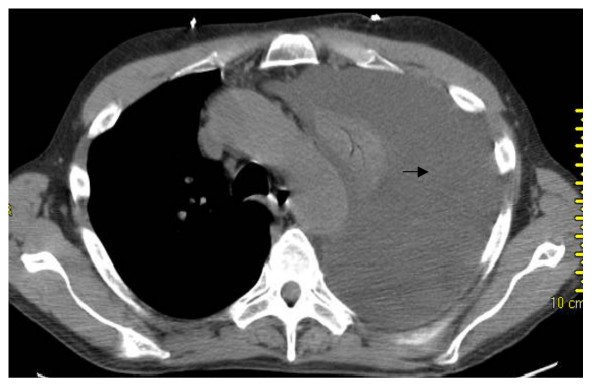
CT chest: massive pleural effusion left side (arrow).
